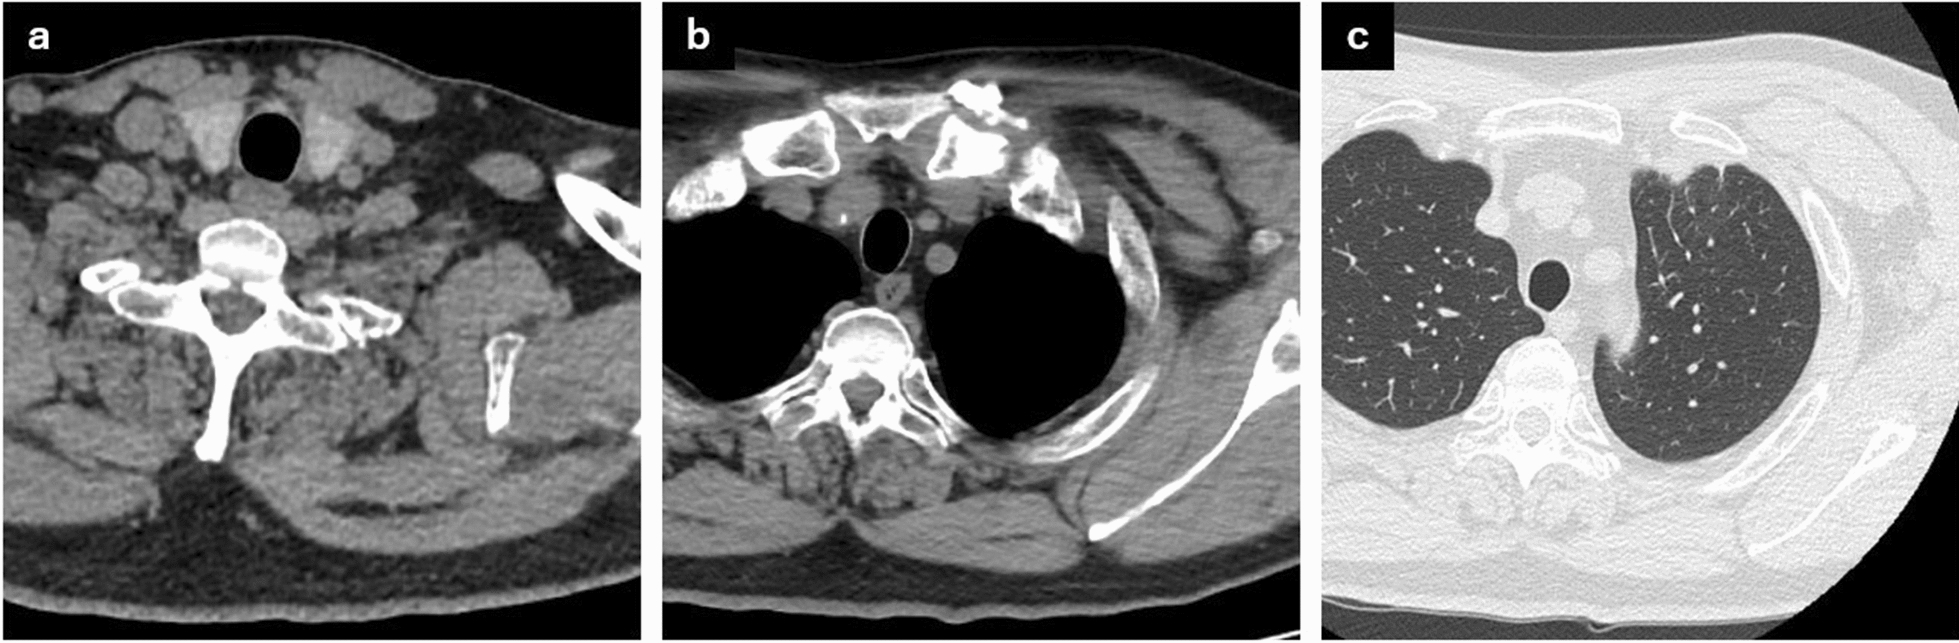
Computed tomography 4 months after completing antibiotic treatment. a Complete resolution of the neck abscess and significant improvement in b the soft-tissue swelling around the left sternoclavicular joint and c lung inflammation
